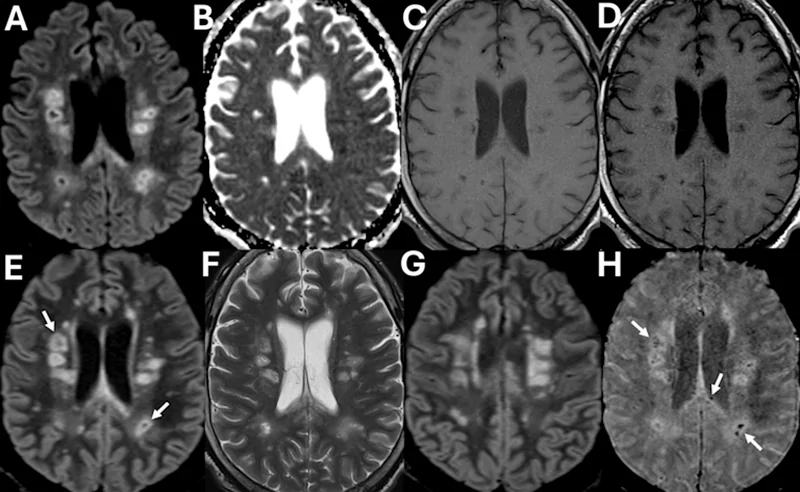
Follow-up brain MRI at 1 month. (
